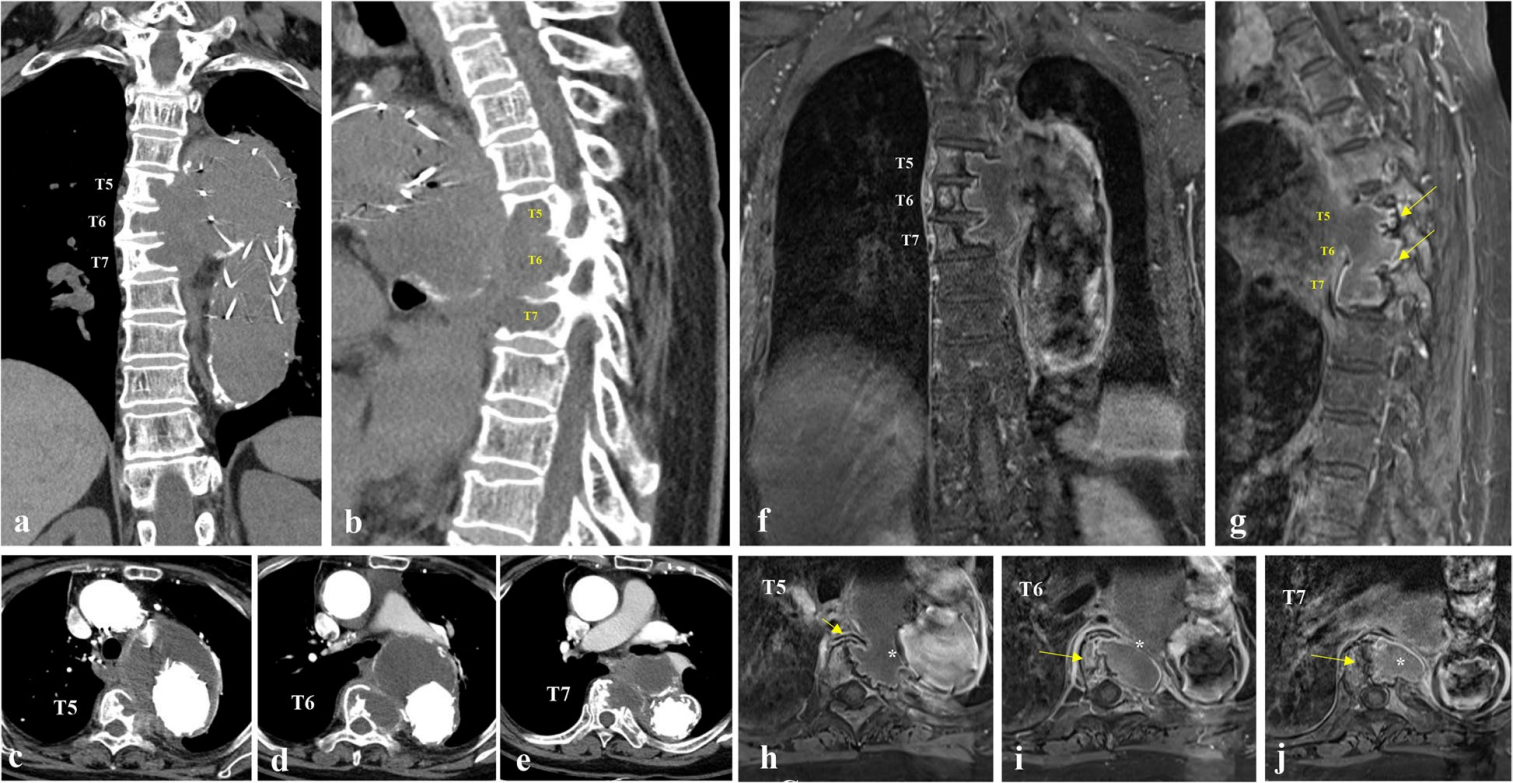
Aggravation of lesion at 3-month follow-up in this case. a–e, Three months later after surgery, follow-up computed tomography of the aorta showed an aggravated perianeurysmal outpouching lesion at the proximal descending thoracic aorta eroding into the T5–T7 vertebral bodies and adjacent left sixth and seventh ribs due to pulsating pressure from the complicated thoracic aortic aneurysm. f–j, Magnetic resonance imaging showed left neural foramen involvement at T5–T6 and T6–T7 and erosion of more than half of the vertebral body at T6 and T7
